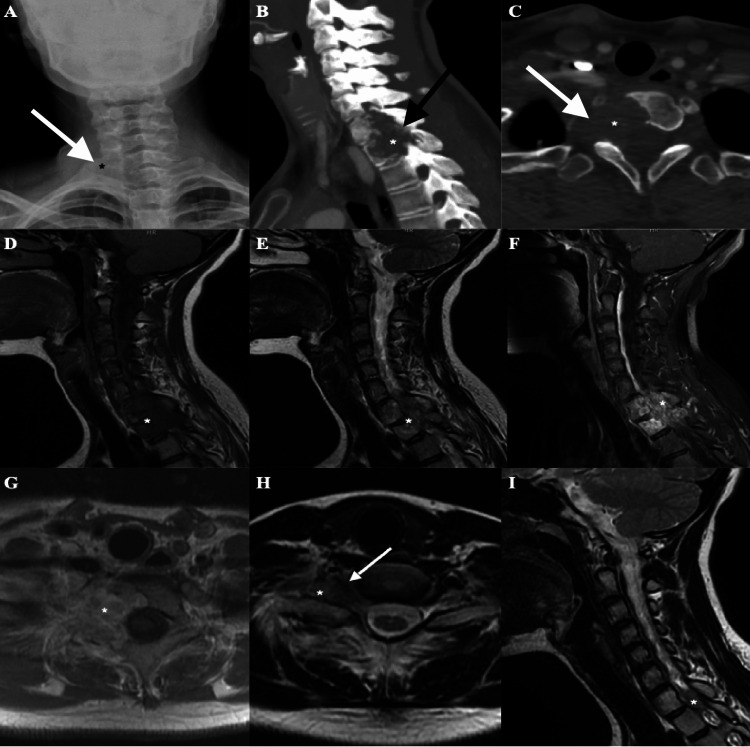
Preoperative images of the patient. (A) The cervical anteroposterior radiograph revealed the presence of an osteolytic lesion that appeared to be casting a shadow on the soft tissue on the right side. (B) The sagittal computed tomography (CT) and (C) axial CT scan revealed that the lesion had infiltrated the C7-T1 region and resulted in osseous destruction. The magnetic resonance imaging (MRI) findings indicated that the lesion exhibited (D) hypointensity on T1-weighted images, (E) hyperintensity on T2-weighted images, and (F) demonstrated significant enhancement on contrast images. The axial MRI images revealed that the neoplasm infiltrated the (G) soft tissue and the (H) right vertebral artery. (I) The patient’s MRI image reveals a tumor with an intradural onset two years ago. *: the tumor. White arrow: right vertebral artery.
